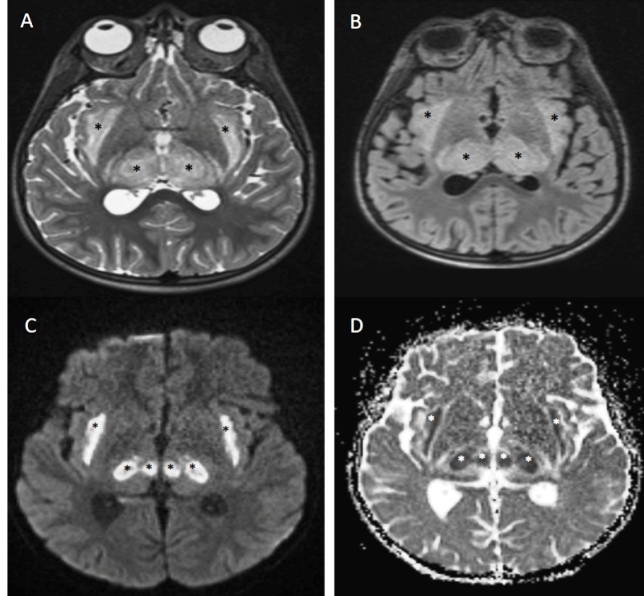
(A) Axial T2-weighted image and (B) axial FLAIR image show the symmetrical hyperintense expansile lesions in the bilateral thalami and external capsules (black asterisks). (C) Axial b-1000 image (black asterisk) and (D) axial ADC diffusion image (white asterisk) show the symmetrical diffusion restriction in the bilateral thalami and external capsules.
